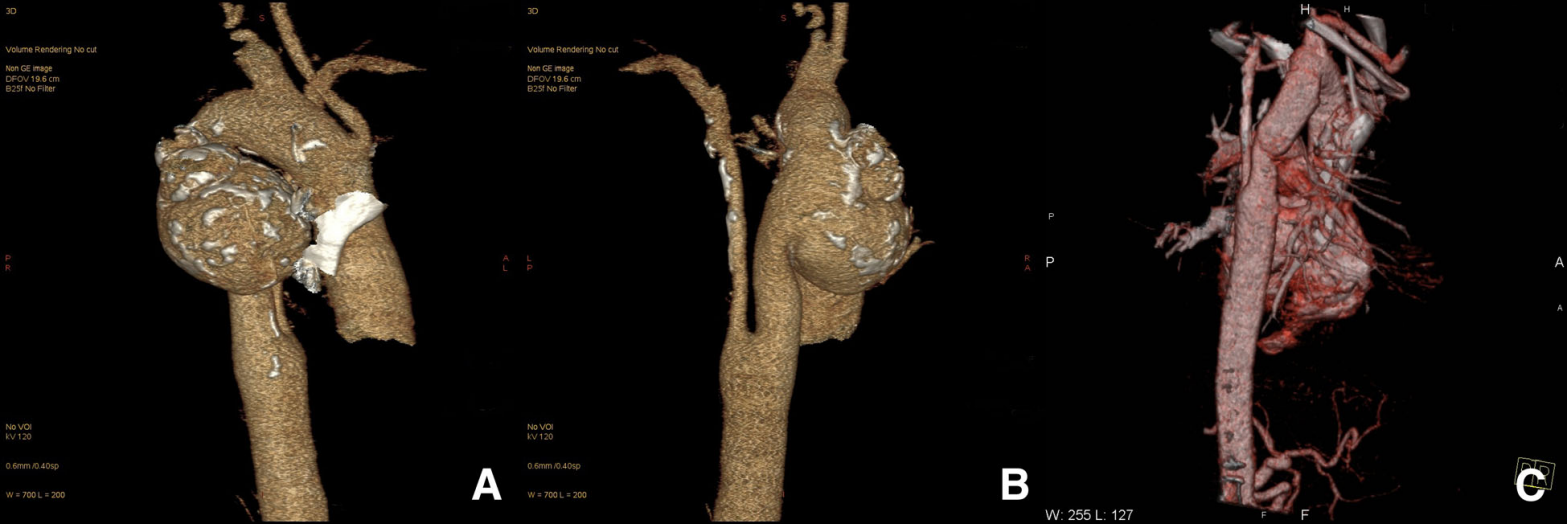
CT depiction of RAA and aneurysmal formation and the descending aorta after surgery (a – dorsal view before surgery, b – descending aorta after surgery)
